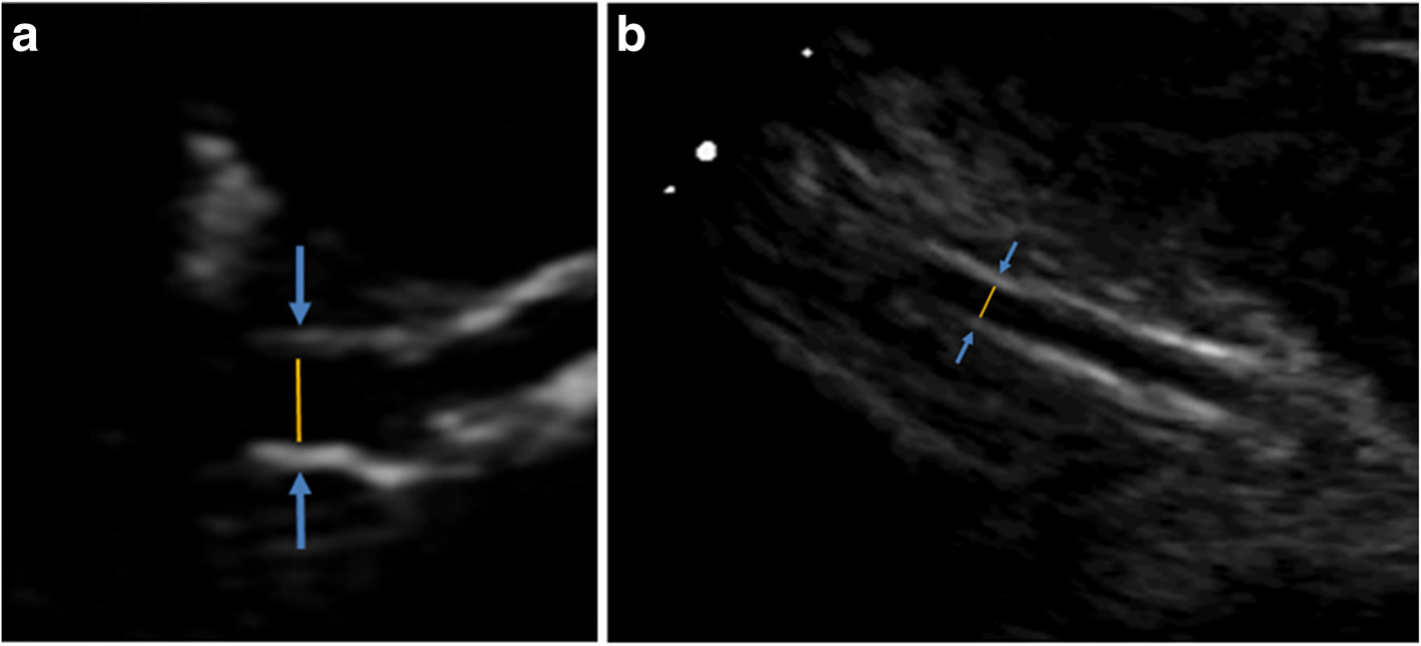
Images of Left Main Coronary Artery (Panel a) and Right Coronary artery (segment 2, Panel b) by trans thoracic echocardiography. Coronary artery walls are visible as two linear echos. Yellow line: luminal diameter of the vessel; blue arrowheads: the external boundary of the vessel
